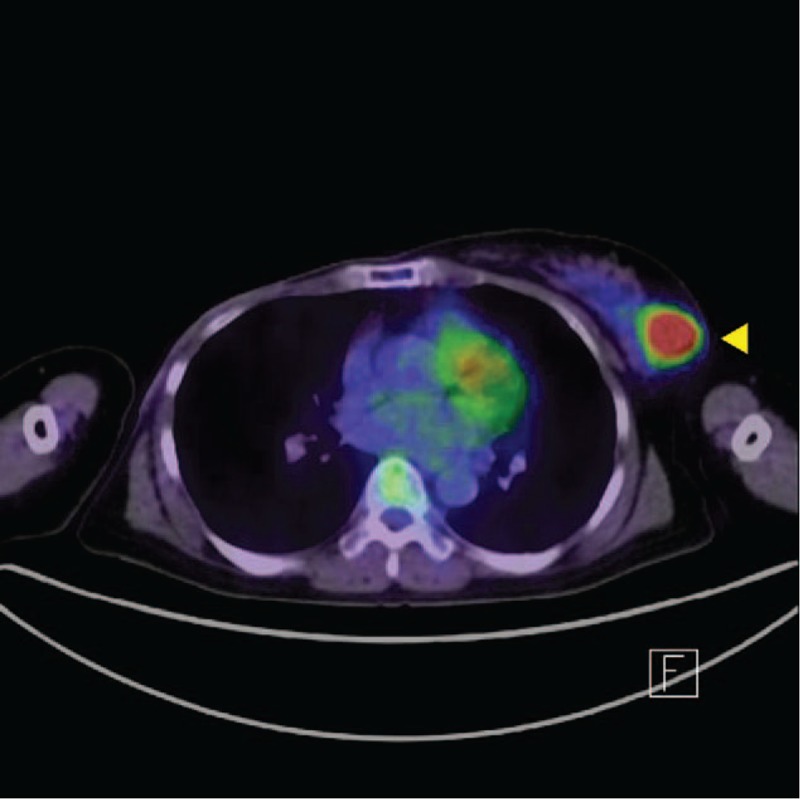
An area of greatly increased uptake was observed in the upper outer quadrant of the left breast (SUV max: 8.2→9.77). A yellow arrowhead in the radiograph indicates the lesion.
